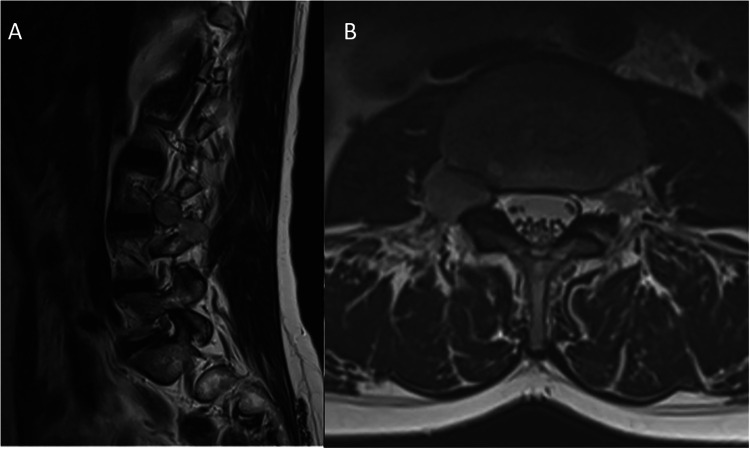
Pre-operative T2-WI sagittal (A) and axial (B) spine MRI. It can be noted the later extension toward the iliopsoas muscle (Asazuma type IIc) which appears as a hyperintense intraforaminal dumbbell shaped lesion.
